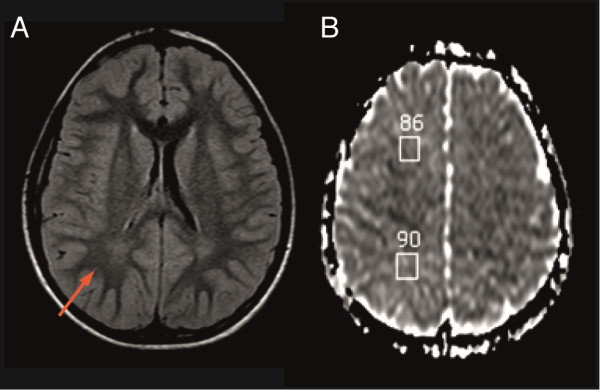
Brain MRI of the patient AUR139_7 at 9 years of age. (A) Axial T2-FLAIR weighted images showed slightly prolonged relaxation times in the periventricular white matter, more prominent in the posterior region (red arrow) with no significant loss of volume. (B) Axial diffusion-weighted images (DWI) showed slightly elevated apparent diffusion coefficient (ADC) values.
